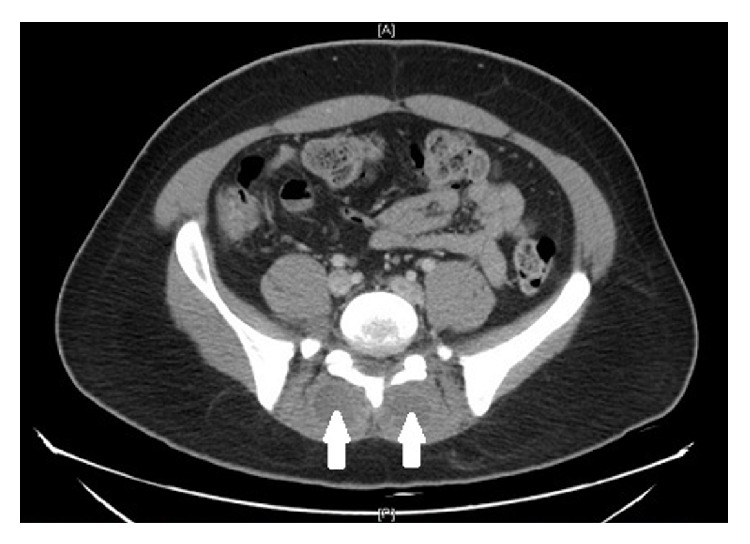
CT of the abdomen with two arrows showing the areas of low-density within the posterior paraspinal muscles.
